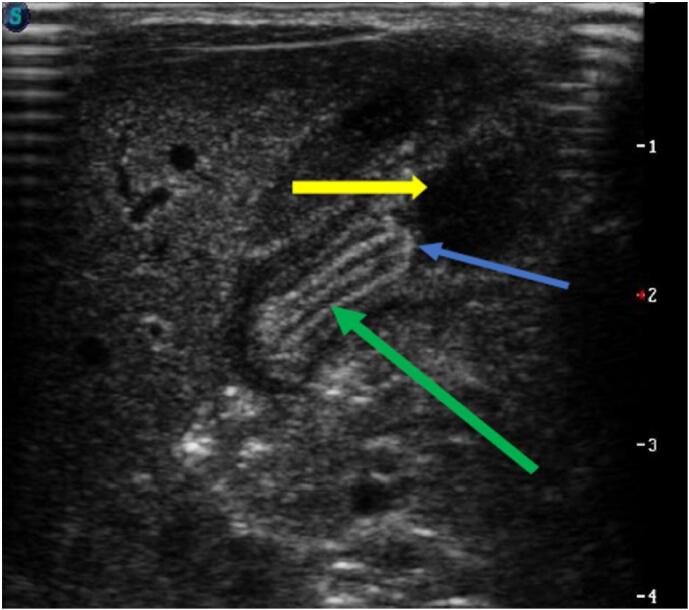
Abdominal ultrasound image of Twin A at 10 days of life, stenosis measuring 5 mm in thickness and 2.1 cm in length (Green arrow) with antral nipple sign (Blue arrow) representing protruding pyloric mucosa and the yellow arrow is the stomach. (For interpretation of the references to colour in this figure legend, the reader is referred to the web version of this article.)
